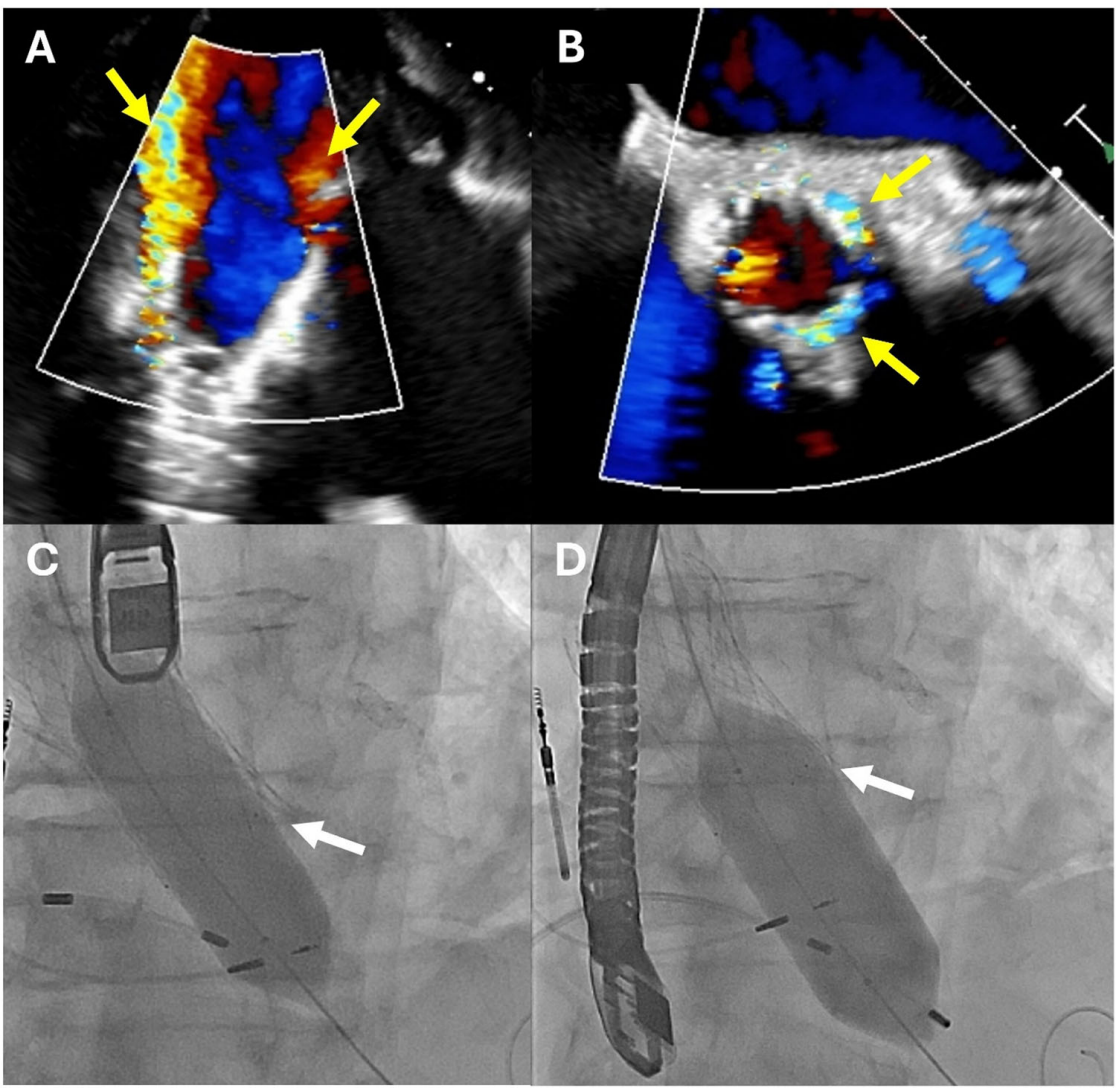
Significant paravalvular leak after deployment of a 26 mm Evolut FX+ valve. TEE color Doppler of the aortic valve in deep transgastric (A) and mid‐esophageal short axis views (B) showed significant PVL in multiple locations (yellow arrows). Post‐dilation with a 22 mm balloon (C) and then a 24 mm balloon (D) did not significantly improve the degree of PVL. A gap remained between the balloon and the inflow of the Evolut valve (white arrows). [Color figure can be viewed at wileyonlinelibrary.com]
